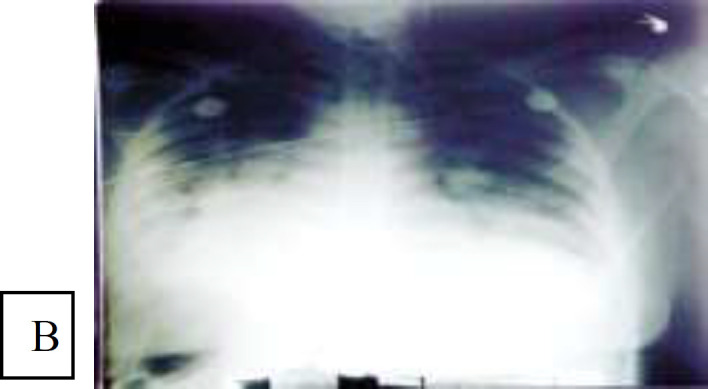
Chest x-rays showing abnormal white patches associated with lung hemorrhage by 3 days interval. The second one (B) showed significant progression of past lesions which dictated bad prognosis
